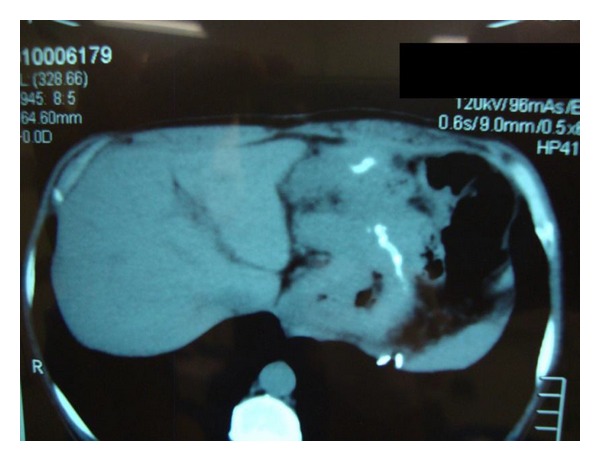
CT scan showing the lesion affecting stomach and with invasion of the left hepatic lobe and the pancreas.
